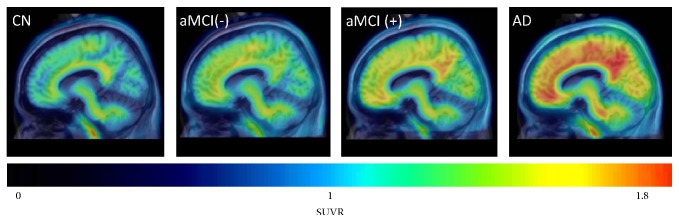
Representative sagittal PET images comparing the standard uptake value ratios (SUVRs) of [^18^F]florbetapir between cognitively normal (CN), amnestic mild cognitive impairment negative for cerebral A*β*, aMCI(−), amnestic mild cognitive impairment positive for cerebral A*β*, aMCI(+), and Alzheimer's disease (AD) patients. Note that higher [^18^F]florbetapir accumulation was especially prominent in parietal, temporal, and occipital gyri. This study also concluded that there was a significant difference between scores on the Mini Mental State Examination for neurocognitive testing and [^18^F]florbetapir SUVRs amid CN, aMCI(−), aMCI(+), and AD subjects ([56], reprinted with permission).
